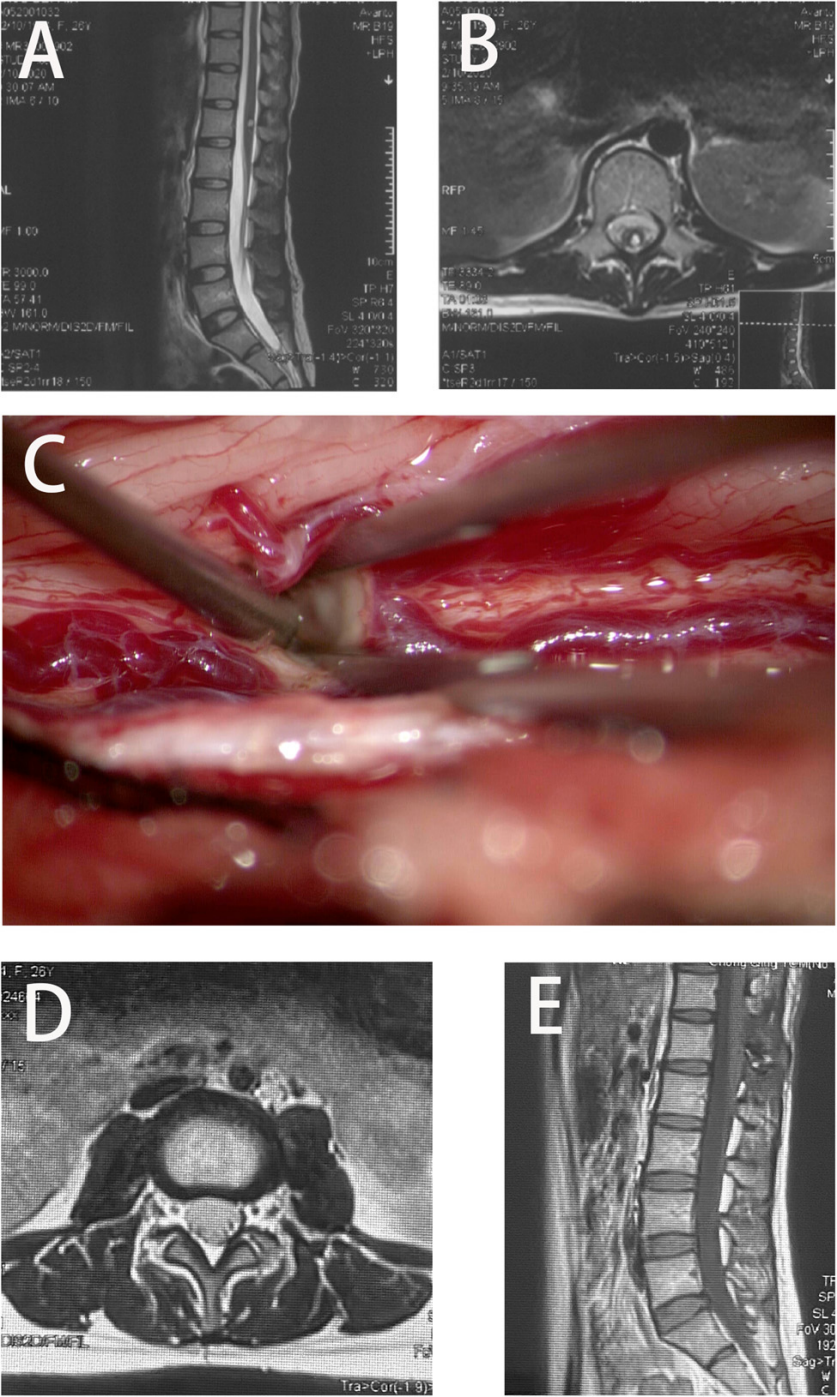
Preoperative MRI (a) and (b), and intraoperative findings (c) showed an SCM at L1 level. Postoperative MRI (d) and (e).
